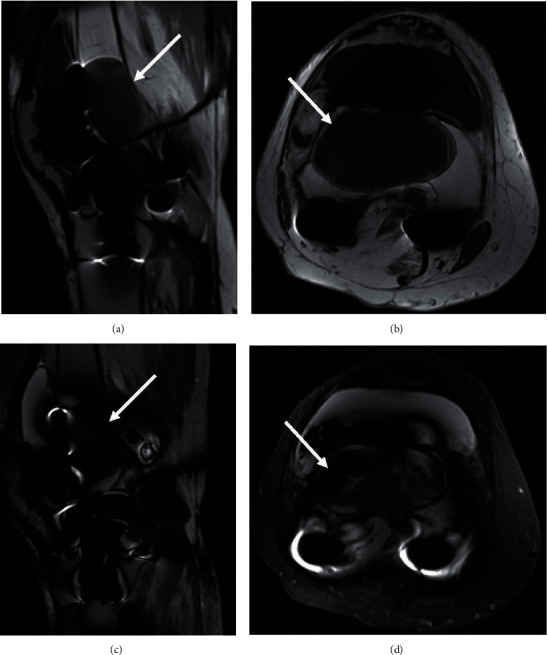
MRI of the right knee with IV gadolinium. In the distal femur, there is low T1 signal in the sagittal (a) and axial (b) views and heterogeneously predominantly low T2 signal in the sagittal (c) and axial (d) views with a sharp rim and thin peripheral enhancement (white arrows) that is in continuity with the prosthesis and typical for osteolysis secondary to particle disease (MRI machine: Titan 3T (Toshiba)).
